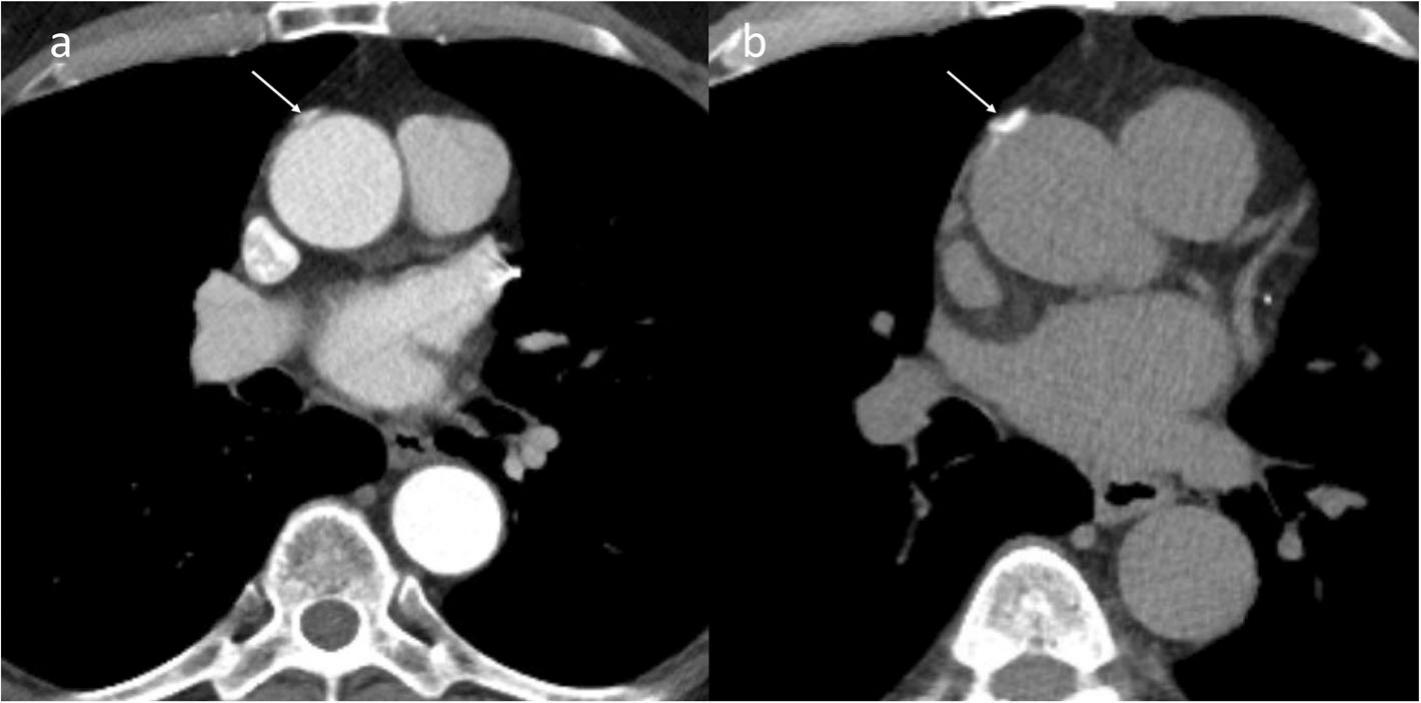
A 89-year-old female patient with acute chest pain. Contrast enhanced axial CT (a) image was suspicious of a small pseudoaneurysm (arrow); however, non-contrast CT (b) image identified the outpouching as surgical pledget (arrow)
